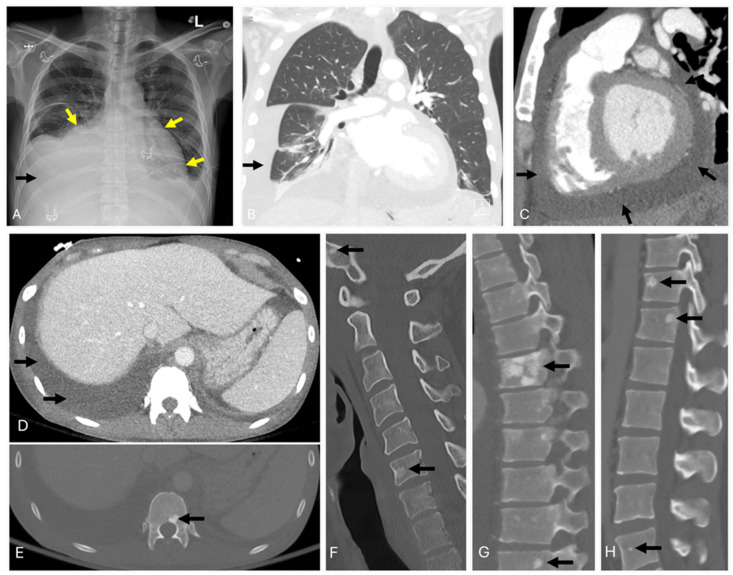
A 40-year-old male with paresthesia. A chest radiograph (A) reveals bilateral pleural effusions, with the right greater than the left (black arrows), pulmonary vascular congestion, and pulmonary interstitial edema. The cardiac silhouette is also enlarged (yellow arrows). These findings are confirmed on the contrast-enhanced chest CT (B). Additionally, a pericardial effusion is seen (C) (black arrows). CT of the abdomen (D) shows anasarca and ascites (black arrows). Sclerotic lesions are seen throughout the axial skeleton (E–H) (black arrows).
